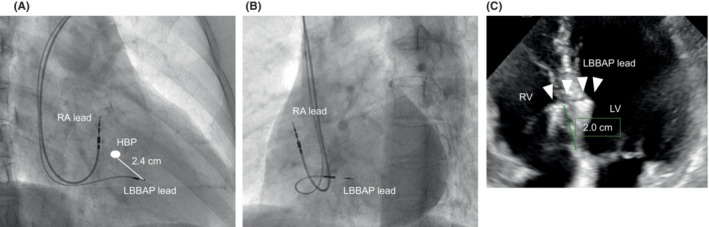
Final fluoroscopic images showing the lead location at (A) an RAO of 30 degrees and (B) an LAO of 50 degrees. (C) Echocardiogram showing the location of the LBBAP lead in the ventricular septum. The LBBAP lead was located approximately 2.0 cm apical from the membranous septum, and the lead tip was near the left ventricular septum endocardium. HBP, His bundle potential; LAO, left anterior oblique; LBBAP, left bundle branch area pacing; LV, left ventricle; RA, right atrium; RAO, right anterior oblique; RV, right ventricle.
